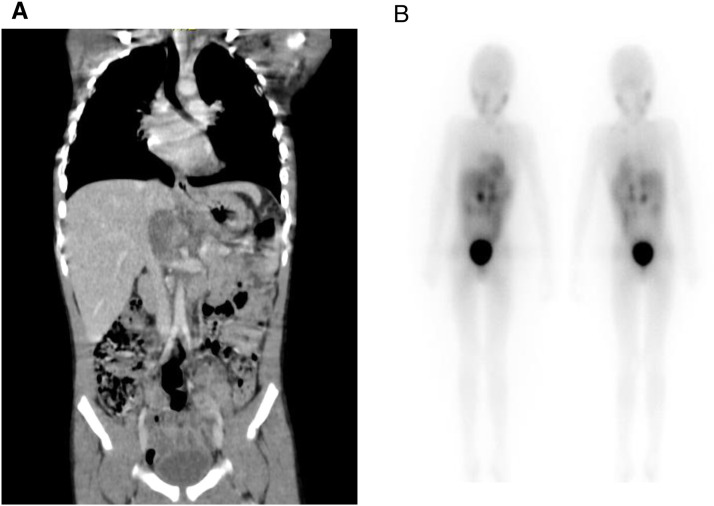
(A) Abdominal tomography after induction with chemotherapy and before the second evaluation, showing a solid retroperitoneal lesion, hypovascularized and with foci of calcification in between, close to the hepatic hilum. (B) MIBG¹³¹ scintigraphy reveals increased uptake in the left infraclavicular lymph node, retroperitoneal region near the periceliac area, hepatic hilum, para-aortic region, inter-aortocaval area, and paracaval lymph nodes.
